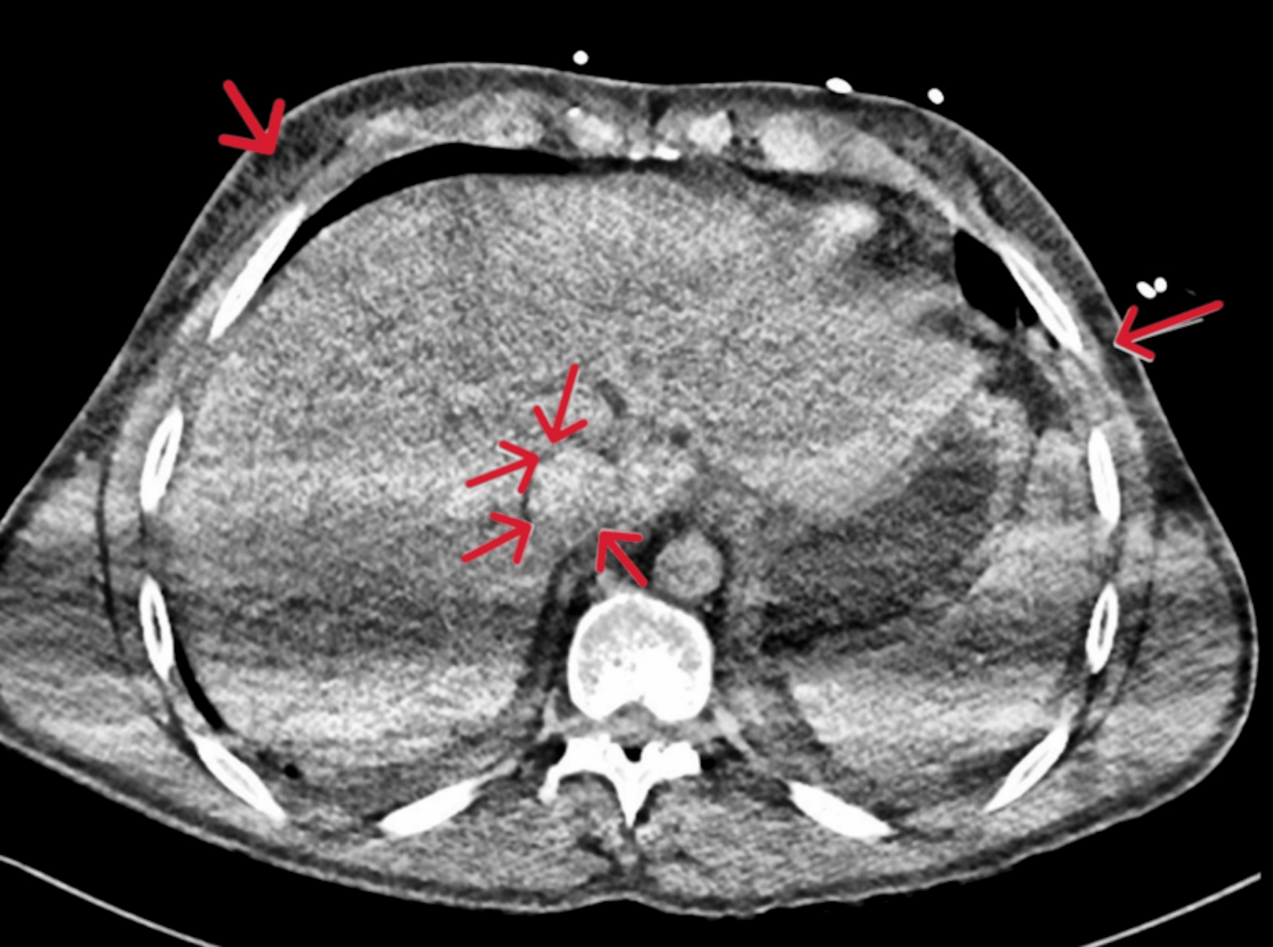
CT abdomen Day 0 transverse section showing diffusely altered attenuation of the liver with prominent hepatic veins and inferior vena cava, suggesting hepatic venous congestion. Generalised oedema of the intra-abdominal and subcutaneous fat. Normal appearance of other solid intra-abdominal organs. No hydronephrosis. Red arrows indicate prominent hepatic veins, the inferior vena cava, and generalised oedema of the subcutaneous fat.
